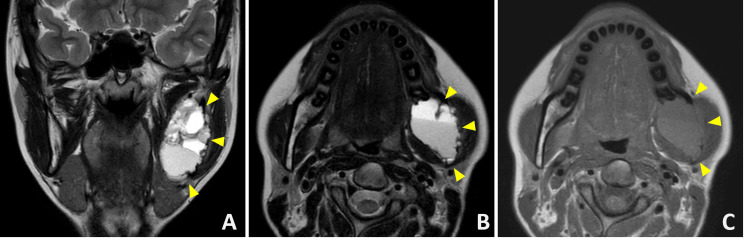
Preoperative contrast-enhanced MRIs (A,B) T1-weighted images showing a multilocular lesion with high signal intensity. (C) T2-weighted image indicating fluid retention within the lesion. The arrows indicate the lesion.
